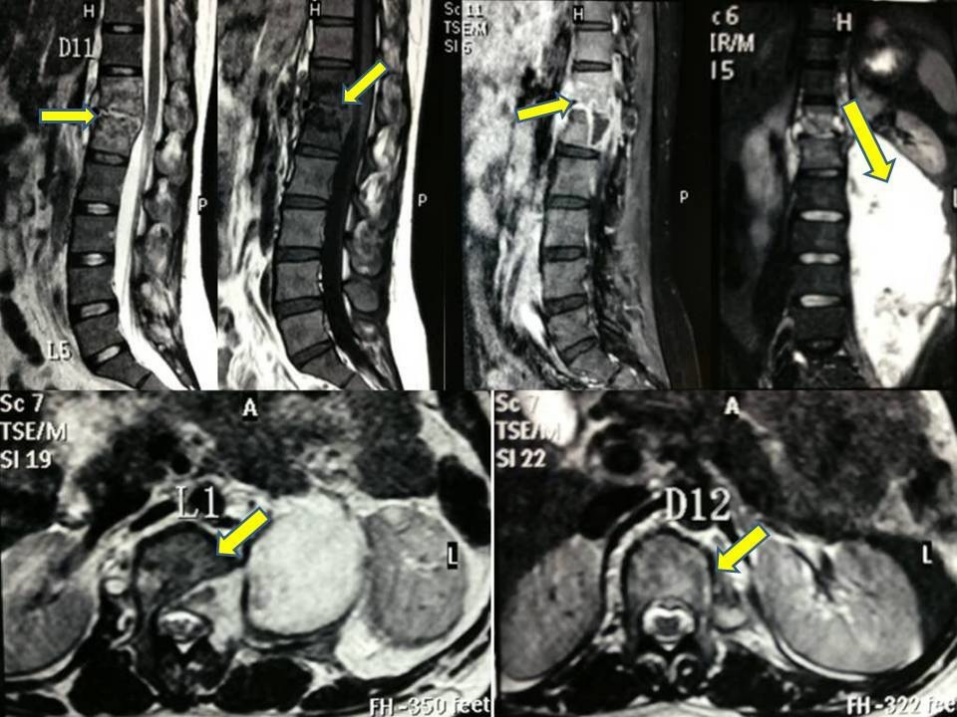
MRI spine (sagittal, coronal and axial cuts) Shows altered marrow signal intensity, hyperintense on T2 and hypointense on T1. There is also a large collection of fluid on the left side iliopsoas region measuring 20.3 cm x 9.2 cm.
